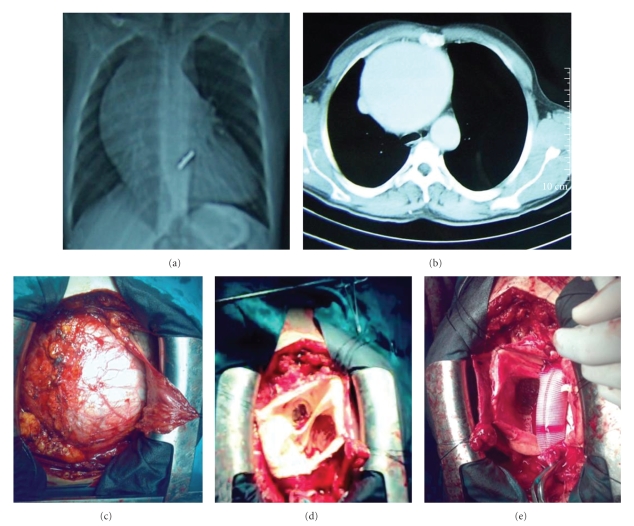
(a) Chest X-ray imaging showing as an aortic enlargement. (b) Spiral thoracic computed tomography imaging showing as a 16-cm aneurysm of ascending aorta. (c) The aortic aneurysm was occupying most of the space in the pericardial cavity. (d) and (e) The aneurysm of the ascending aorta was resected and implanted with a woven graft associated with the left and right coronary artery button implantation.
